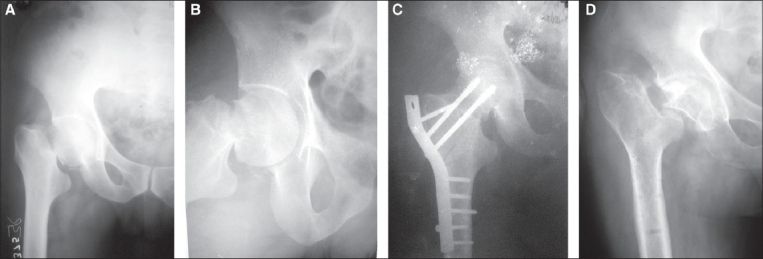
Nonunion following primary valgus osteotomy and osteosynthesis. (a) and (b): Pre-operative antero posterior and Lateral views showing intracapsular fracture neck femur. (c): Postoperative X-ray antero posterior view showing the screws' length is short. This fracture did not unite and the screws cut through the superolateral aspect of the femoral head. (d) X-ray antero posterior view showing un-united femoral neck fracture with healed osteotomy (after 1 year of the index procedure the implants were removed. Patient could walk unaided with painless limp and refused further surgery)
